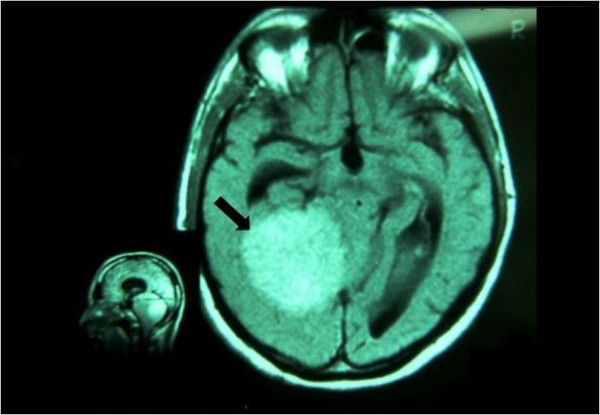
Magnetic Resonance Imaging (MRI). The patient's MRI scan shows a large meningioma, 8 cm in diameter (arrow) with lateral displacement of the brainstem.
